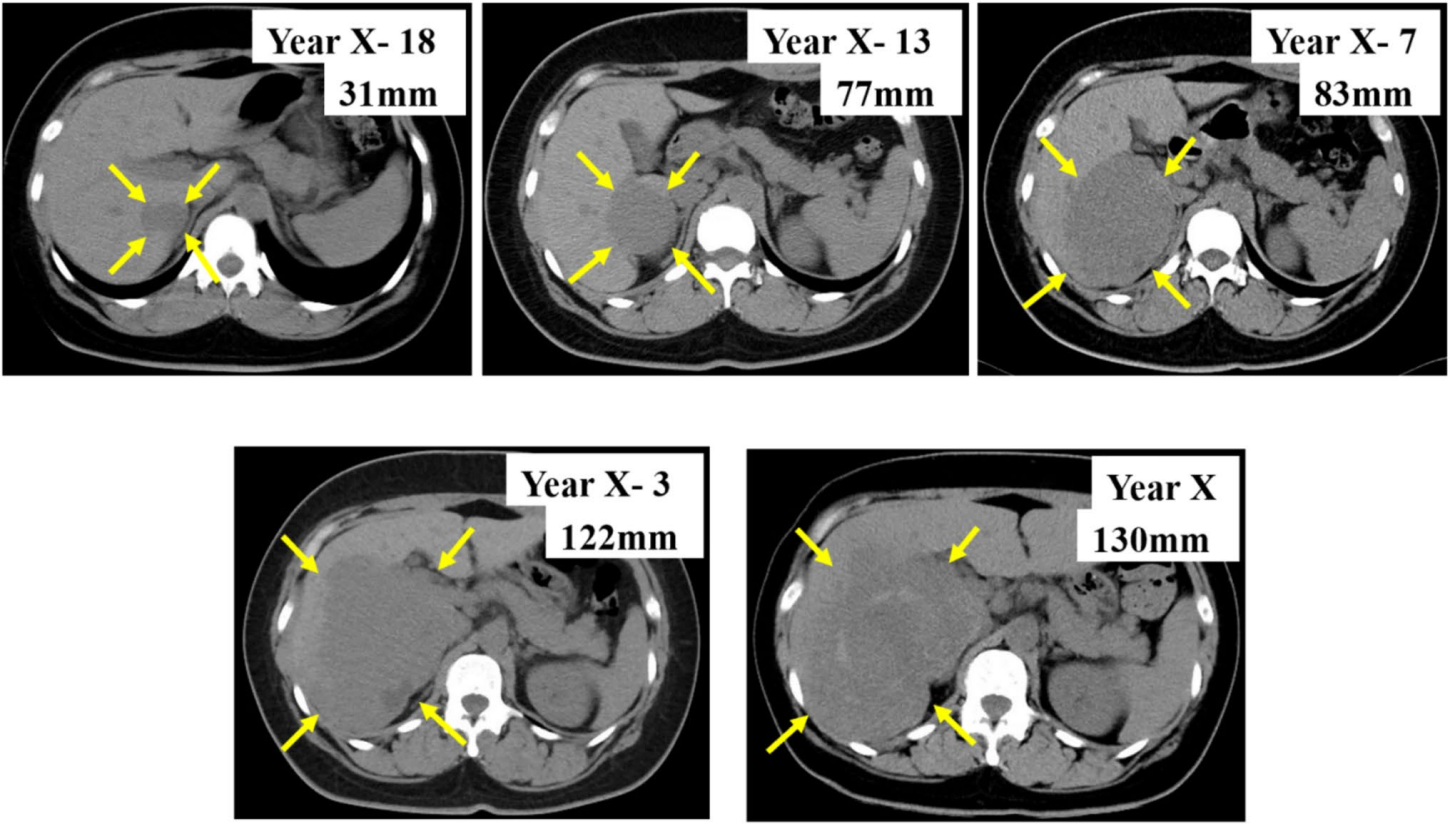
Abdominal CT. The hepatic hemangioma in the posterior zone of the right lobe of the liver showed an increased transition from 31 mm to 130 mm over the course of 18 years. The yellow arrows indicate the edge of hepatic hemangioma. *CT* computed tomography
